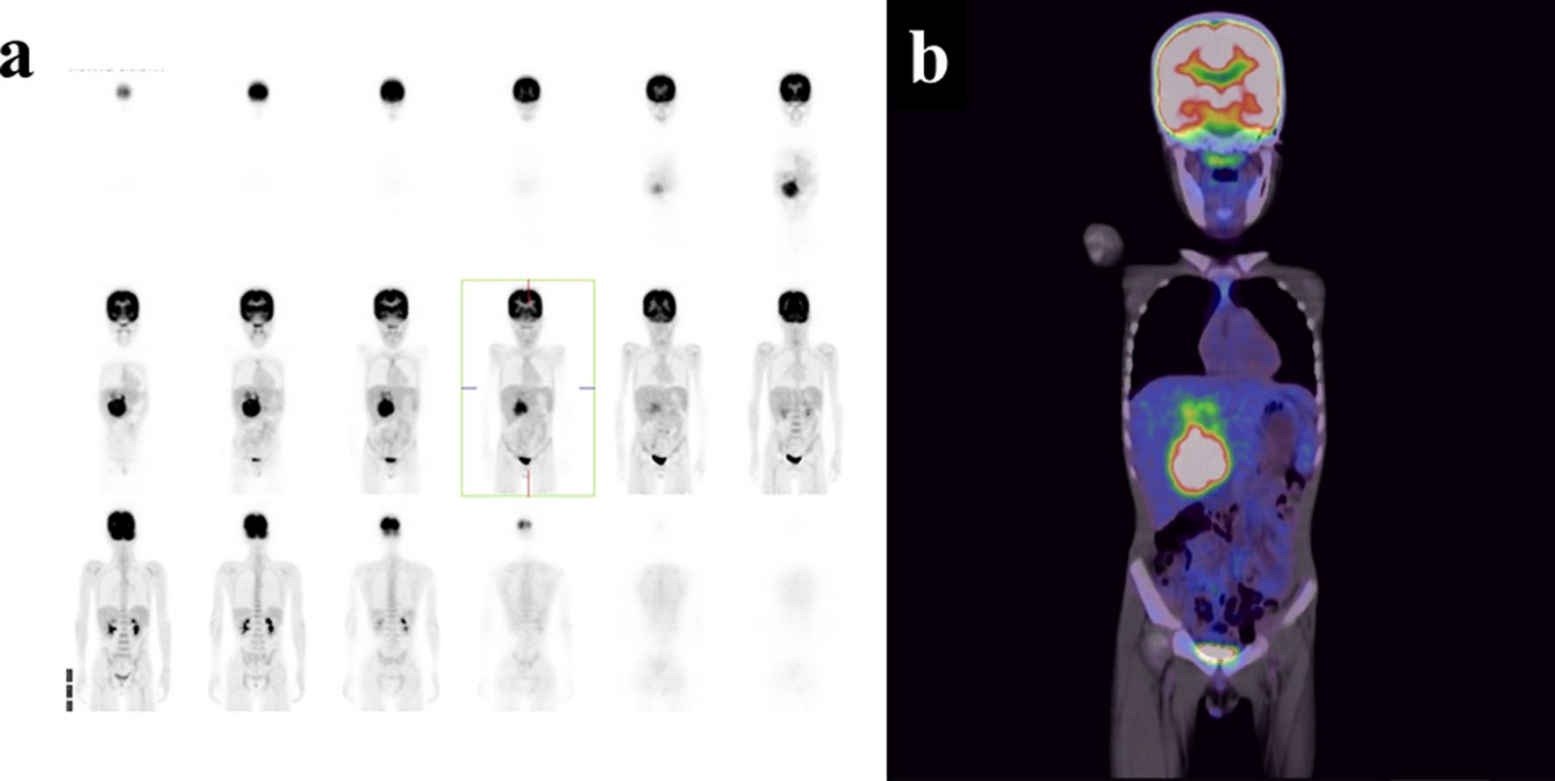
FDG-PET findings. FDG-PET shows the tumor to have a high FDG uptake (SUVmax: 8.8) (b) with no signs of distant metastasis (a)
